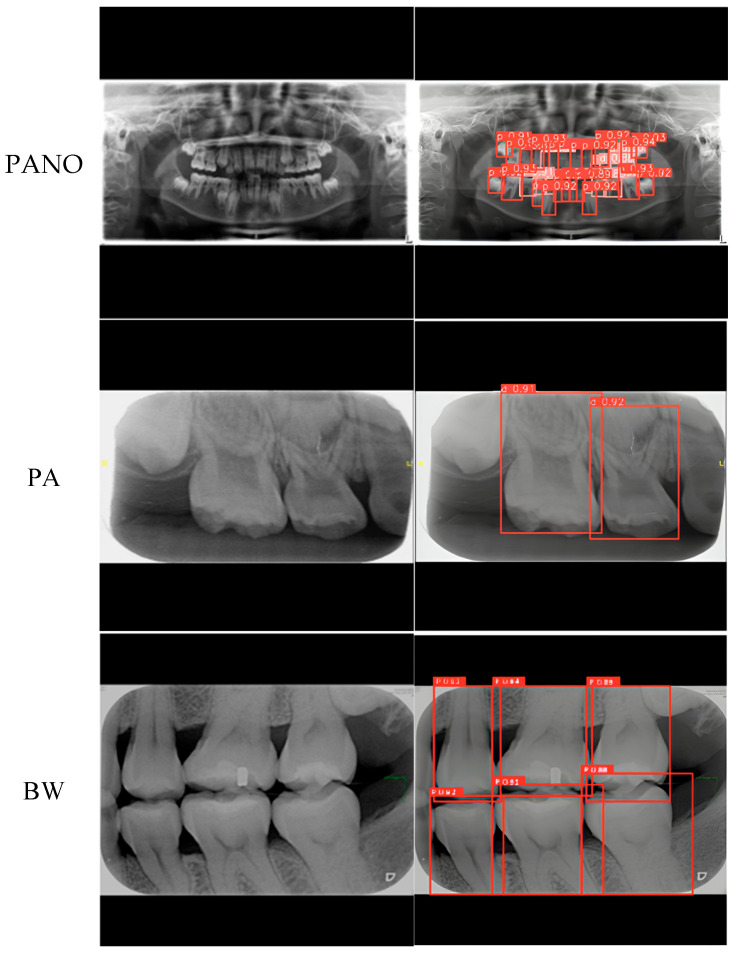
The results of the localization of various dental X-ray images in this study.
